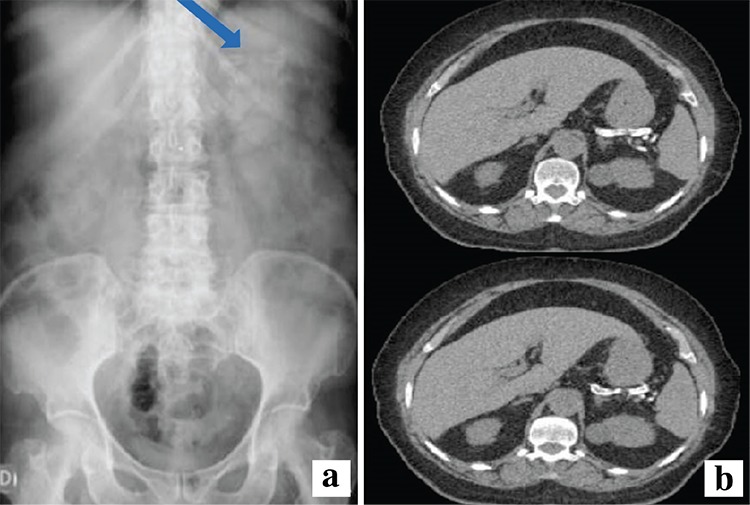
Abdominal plain radiography showing radiopaque tortuous parallel lines with a tubular aspect on the left upper quadrant (a). Computed tomography images confirming the isolated calcified splenic artery (b).
